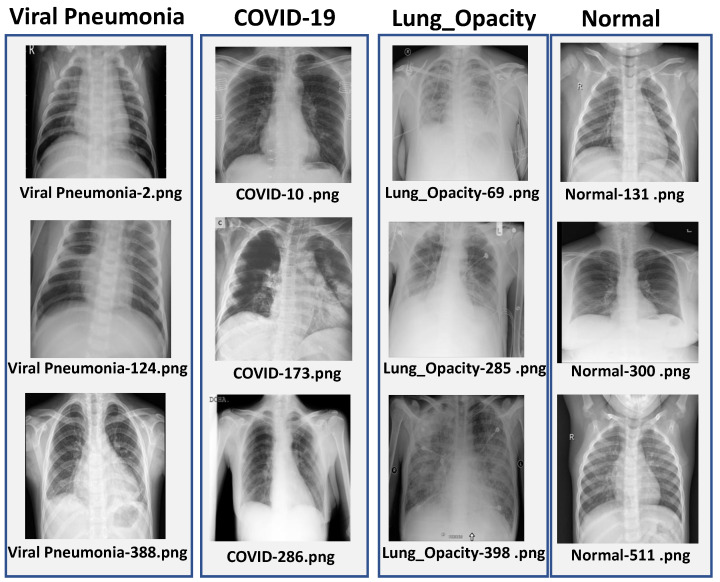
Sample chest X-ray images from each class in the dataset: COVID-19, Viral Pneumonia, Lung Opacity, and Normal.
